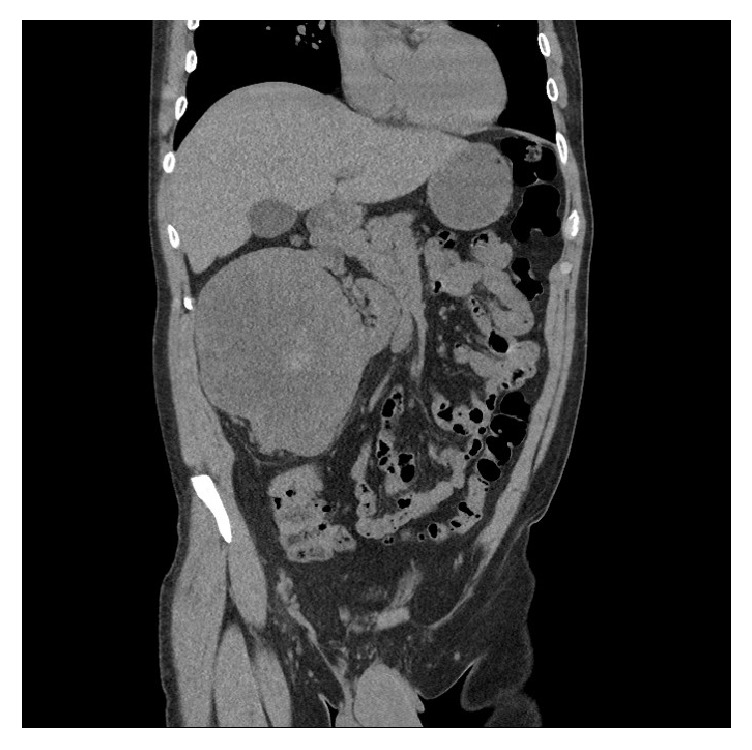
Computed tomography without contrast demonstrating a large heterogeneous right renal mass.
